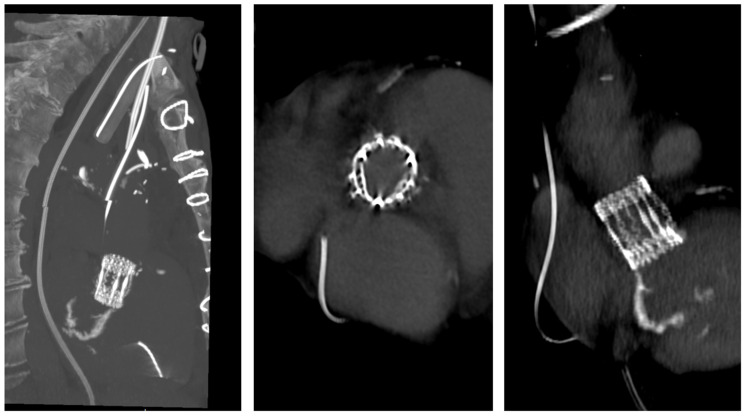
Post-operative Three-dimensional CT reconstruction demonstrating the Perceval sutureless valve in optimal position.
